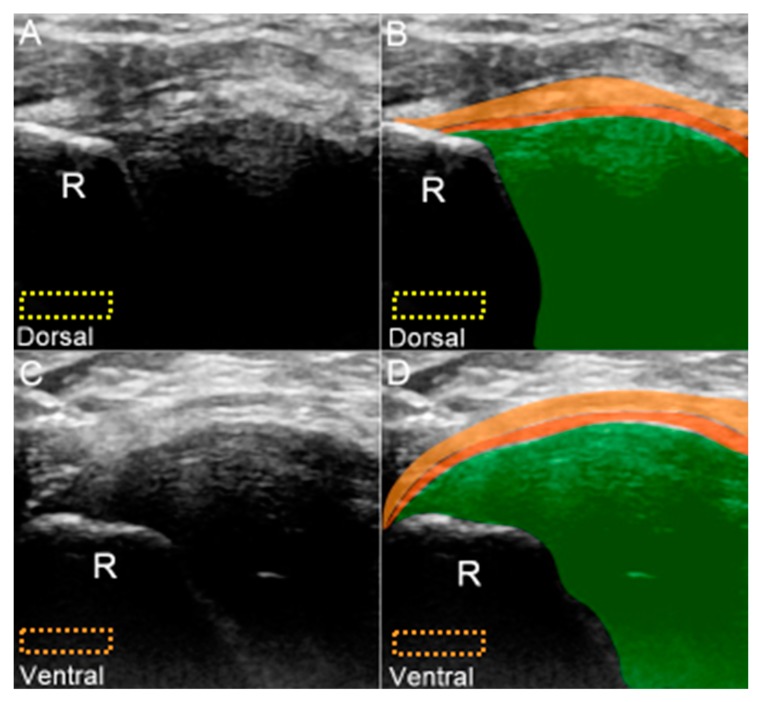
(A) US and (B) superposed US images of the dorsal radioulnar ligament. (C) US and (D) superposed US images of the ventral radioulnar ligament. The different color dashed rectangles are in accordance with the transducer positions in Figure 3. US: ultrasound; R: radius. Superficial limb of the radioulnar ligament *(light brown shade)*, deep limb of the radioulnar ligament *(dark brown shade)*, and articular disc *(green shade)*.
